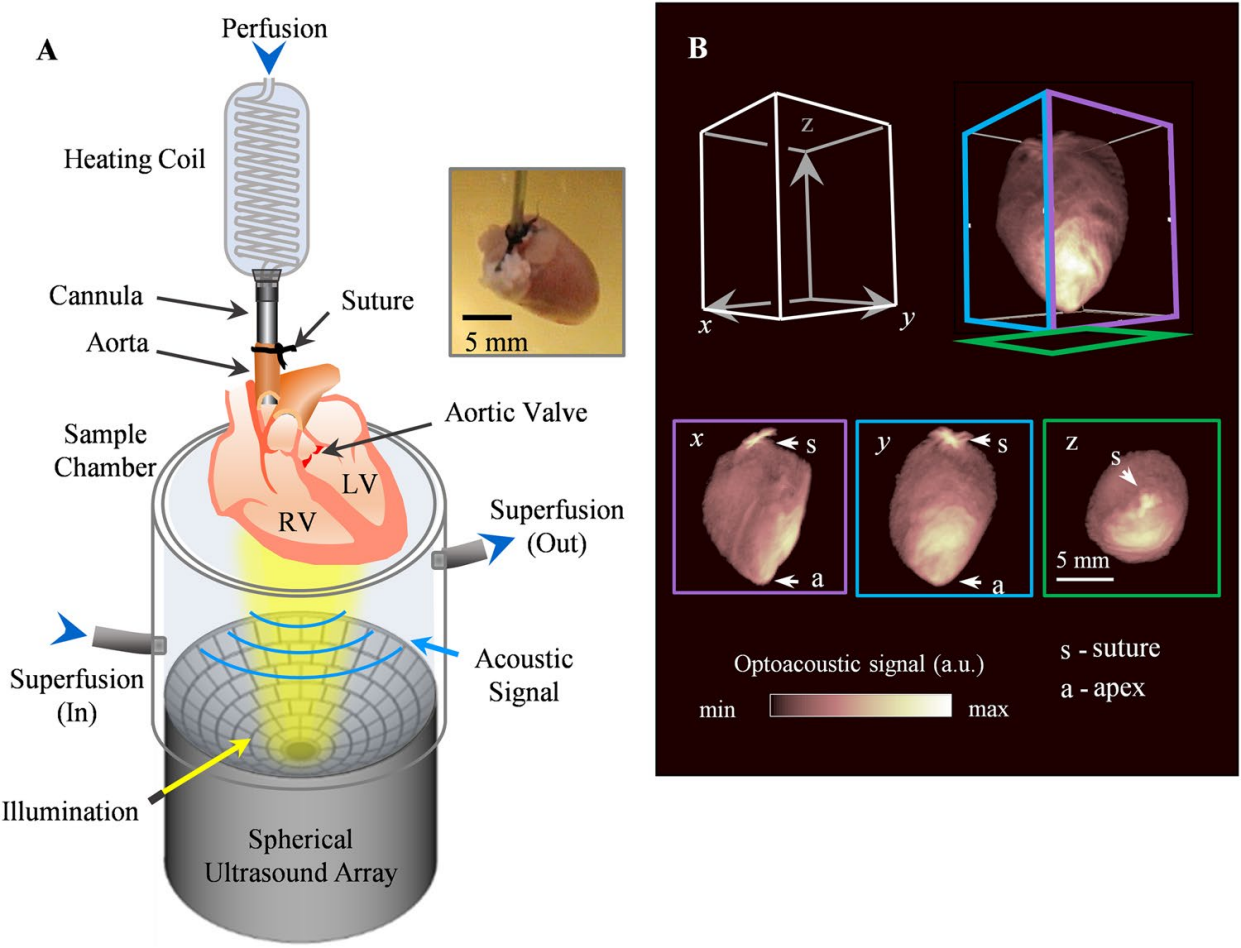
Imaging setup of real-time volumetric optoacoustic imaging of Langendorff-perfused heart. (A) The heart was attached to the output of the heating coil and perfused through the cannula. It was then suspended into the sample chamber from above. The perfusate filled the volume of the chamber, with input and output ports facilitating flow for sample superfusion. Short (10 ns duration) light pulses at 800 nm wavelength and 100 Hz pulse repetition frequency were delivered onto the heart from below while a spherical matrix ultrasound array was used to collect the generated optoacoustic responses. For each light pulse, a three-dimensional optoacoustic image was rendered, shown in (B), along with the maximum intensity projections (MIP) in *x*, *y*, and z dimensions. The Langendorff-perfused heart could be seen in its entirety, and the indicated features are the suture (s) and heart apex (a).
